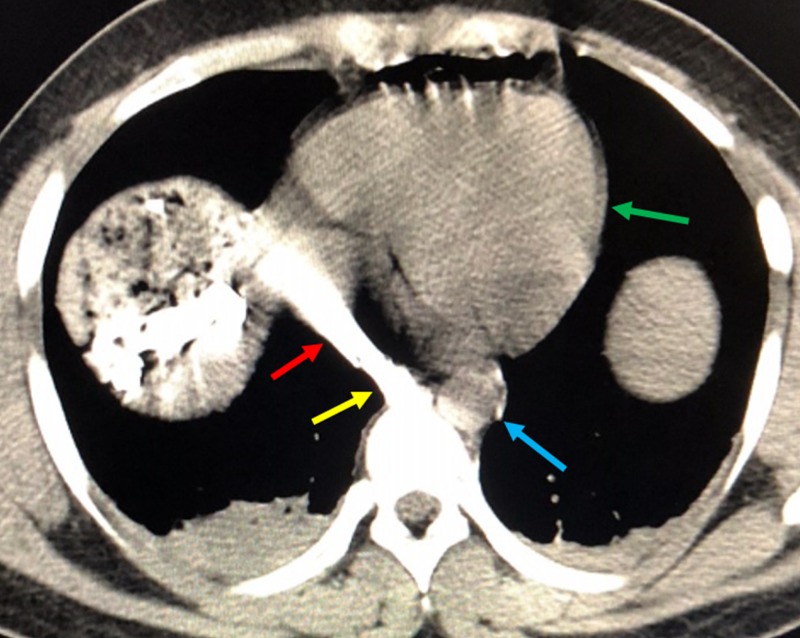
Initial Non-Contrast Axial Computed Tomography of the Chest The bullet is seen in the inferior vena cava (yellow arrow). There is significant streak artifact from the metallic shrapnel (red arrow) adjacent to the intact aorta (blue arrow). The green arrow delineates the heart.
